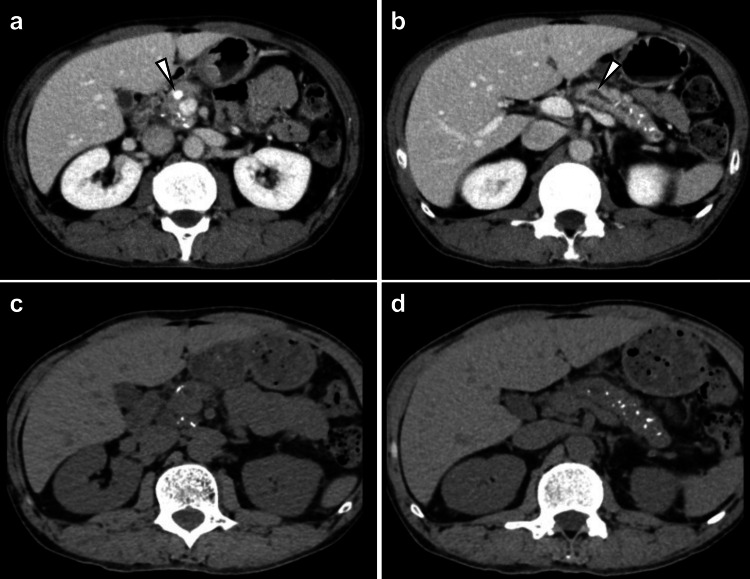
CT images before and after ESWL (a) Multiple calcifications were observed in the pancreatic head. The presence of a 10 mm long pancreatic ductal stone was confirmed in the main pancreatic head (white arrowhead). (b) Multiple calcifications were also observed in the pancreatic tail. The MPD was dilated on the distal side of the pancreatic stone (white arrowhead). (c) After ESWL, the pancreatic stone was destroyed. (d) Dilation of the distal pancreatic duct was relieved. CT: computed tomography, ESWL: extracorporeal shock wave lithotripsy, MPD: main pancreatic duct
